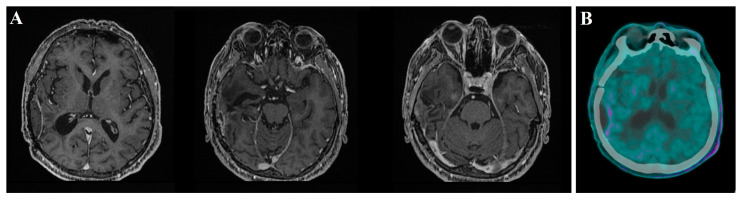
One-year follow-up postoperative T1-weighted brain MRI axial sequences with gadolinium reveal locoregional meningeal enhancement in the absence of neoangiogenesis signs on perfusion study. The previously observed right temporo-mesial lesion shows no enhancement and exhibits no signs of neoangiogenesis in the perfusion study (A). The 11C-methionine PET/CT brain examination documents a mild, heterogeneous tracer accumulation along the right temporo-parietal surgical cavity margin, with no additional abnormalities or asymmetries detected in the remaining brain areas. This is indicative of the absence of cerebral disease (B).
